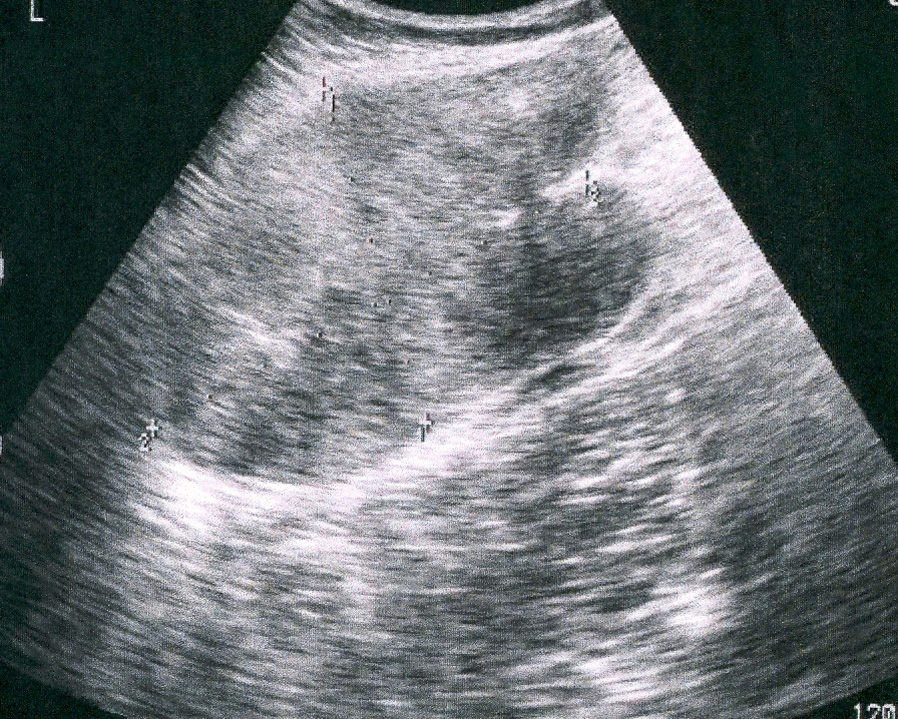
Ultrasound image showing a large mass of heterogeneous echogenicity with smooth, sharply defined margins.
